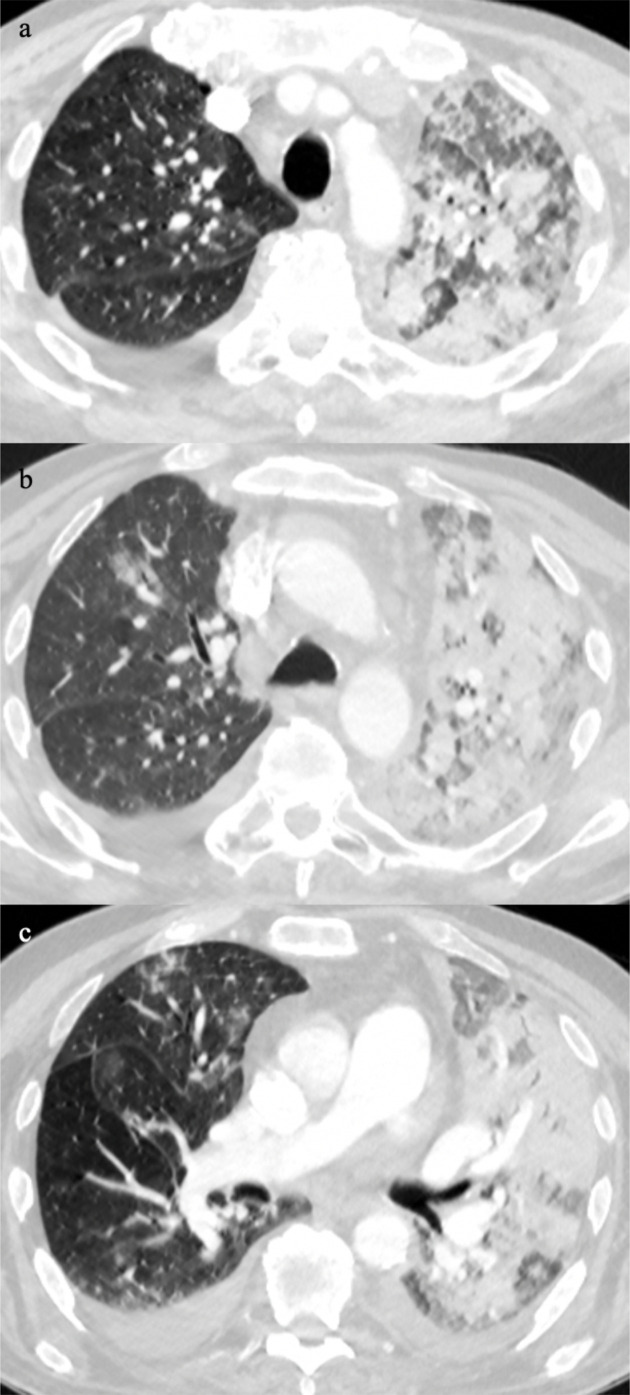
Chest CT showed predominately bronchocentric ground glass opacification in the right upper lobe and middle lobe with bilateral pleural effusions and dense consolidation on the left (5a-c).
